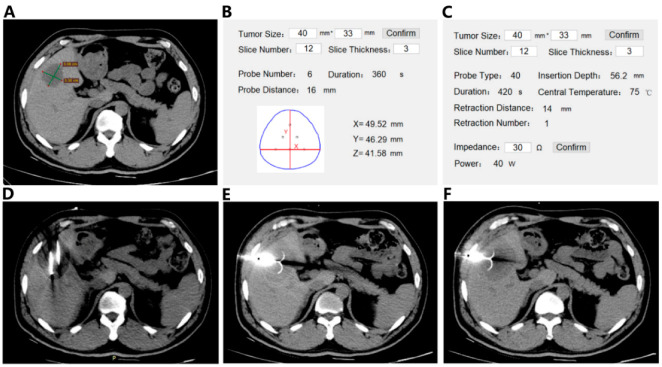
Operational procedure of the multi-mode thermal therapy: a representative case. (A) The axial CT imaging showing a 40 mm liver tumor in the patient; (B) the plan for freezing; (C) the plan for heating; (D) freezing process using seven cryo-probes (CryoHitTM, GALIL Medical, Israel). The low signal area near the cryo-probes indicating the formation of ice ball; (E) the first step of radiofrequency ablation (RFA), the probe with 4.0 cm active tip (MedSphere, Shanghai, China) inserted to the bottom of the tumor; (F) the staged retreat heating process to make the heating range cover the entire tumor.
